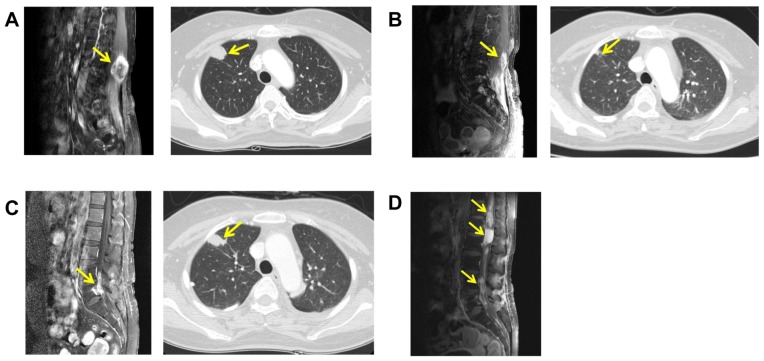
A 46-year old women with DFSP at paravertebral site at the level of L3–4 and RUL zone of chest. Lumbar spine MRI and chest CT were taken. A, before initiation of treatment with imatinib. B, after 4 months treatment with imatinib. C, after further 2 months treatment with imatinib and 3 months treatment with higher dose imatinib. D, at the time of disease progression with leptomeningeal metastasis.
